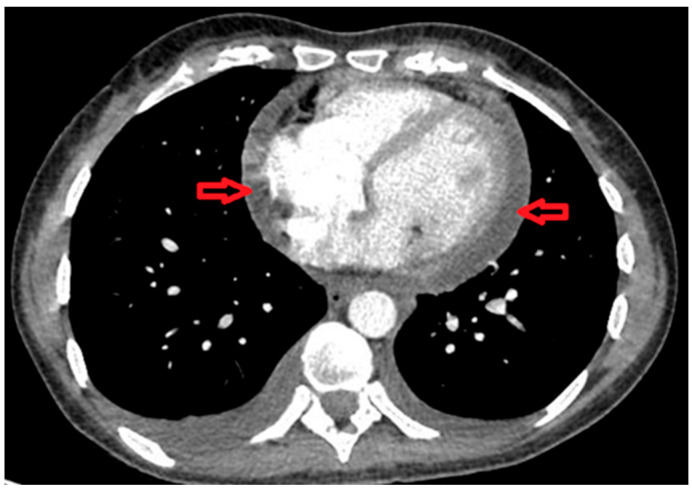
CT scan shows evidence of pericardial effusion (red arrows).
